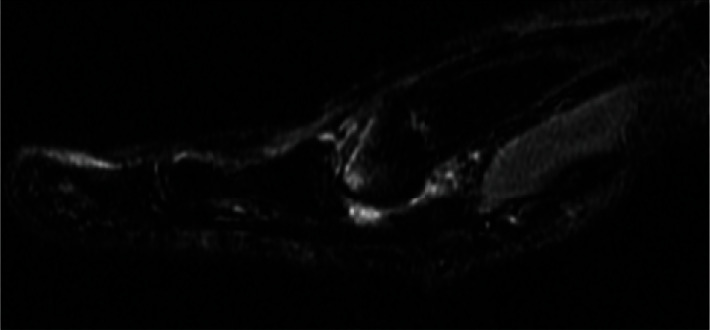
Preoperative right foot MRI performed 14-month status postdorsal cheilectomy demonstrating 50% loss of the first metatarsal head.
